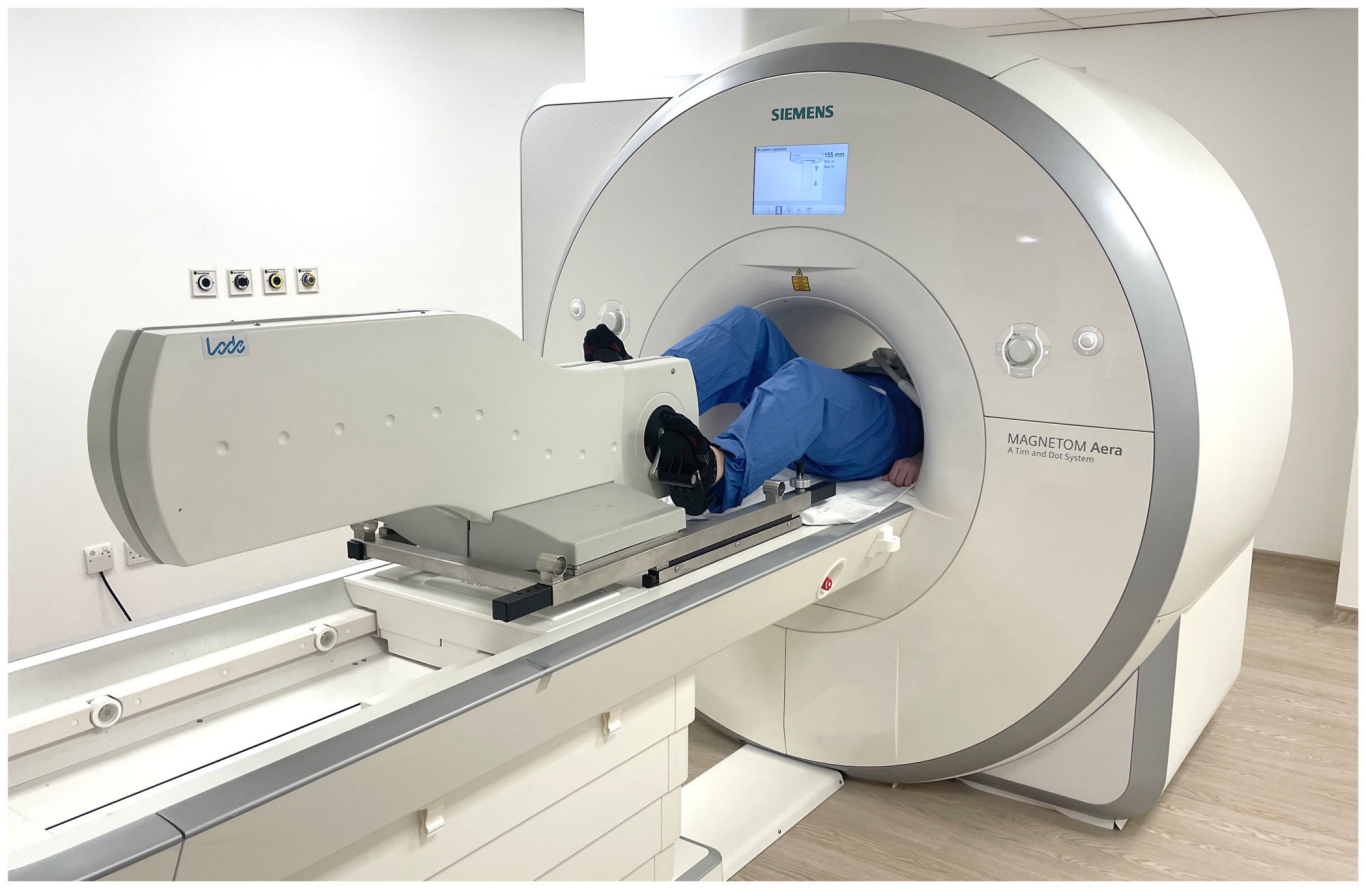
Pedal ergometer. Positioning of the bed-mounted MRI-conditional pedal ergometer allowing participants to exercise while at isocentre (hand holds not shown). An electro-magnetic braking system allows a maximum peak load of up to 300W. An external control unit displays multiple ergometry parameters in real time.
